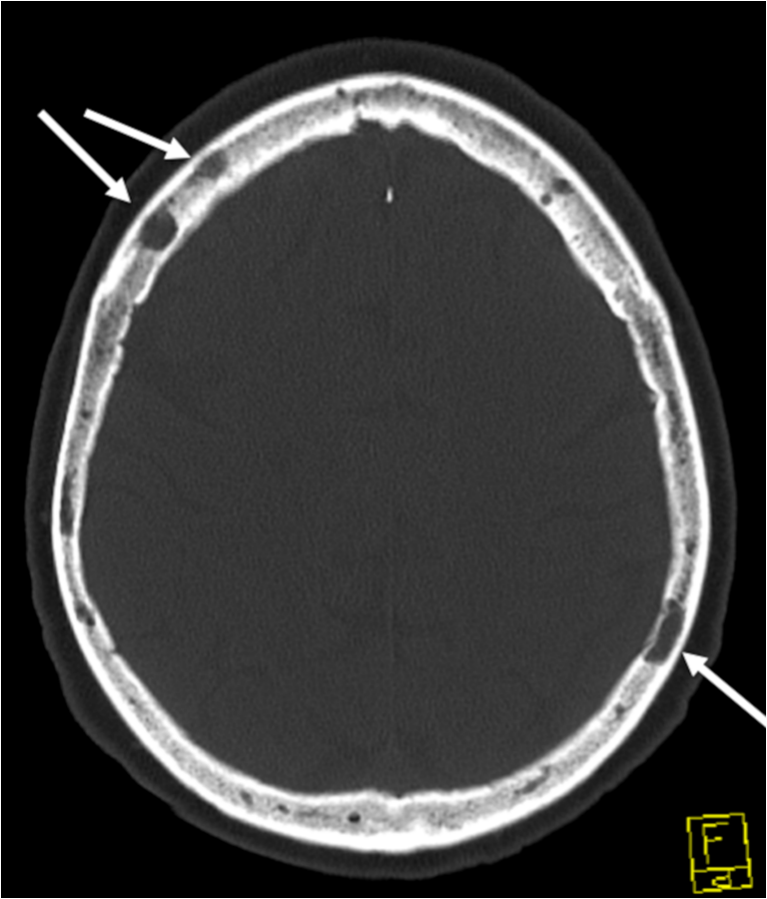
Axial view of a CT scan of the head in the bone window. The white arrows mark typical punched-out osteolytic lesions in the skull of a 48-year-old female patient with multiple myeloma
